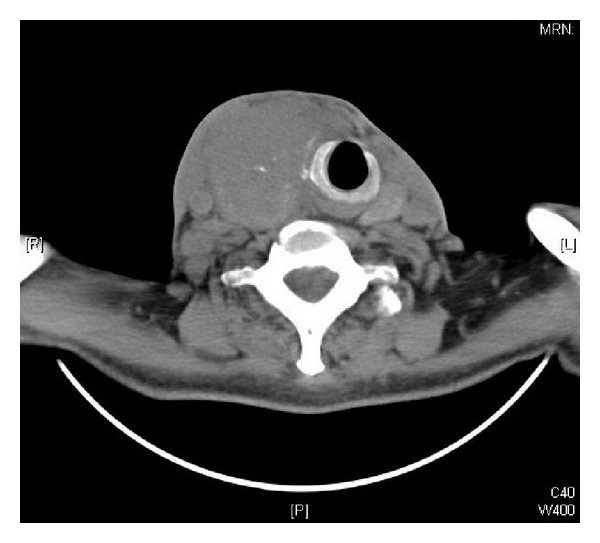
Computed tomography of neck showing left thyroid mass of size 8 × 7.5 cm.
